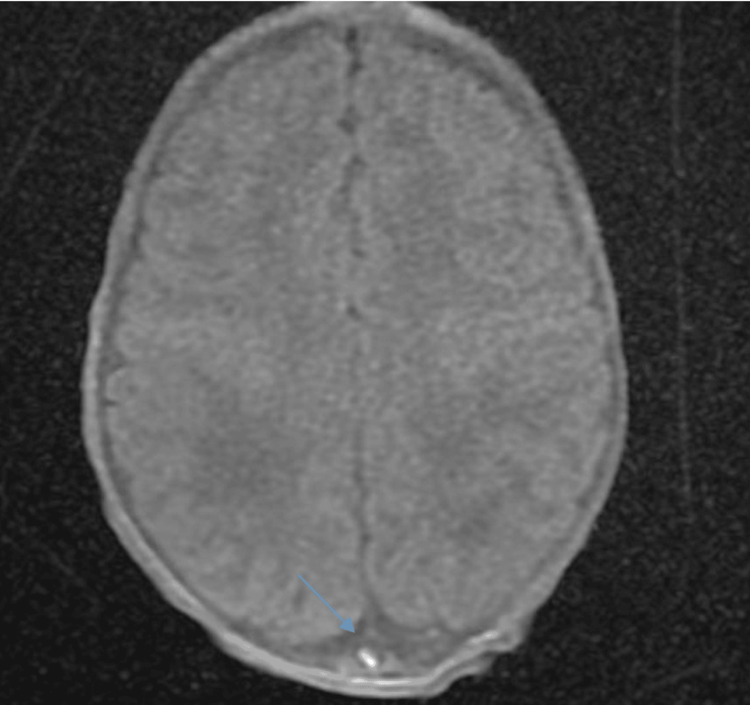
Brain MRI in axial T1 sequence without gadolinium injection showing a hyperintensity in the lower part of the superior sagittal sinus (blue arrow) MRI: magnetic resonance imaging
